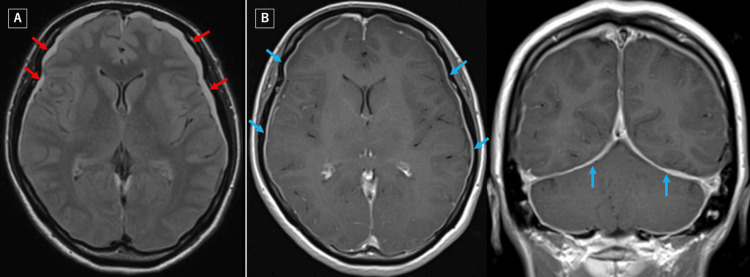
Fluid-attenuated inversion recovery (FLAIR) image shows bilateral subdural effusion (red arrow) (A). Gadolinium-enhanced T1-weighted images (axial and coronal views) show pachymeningeal enhancement (blue arrow) (B).
